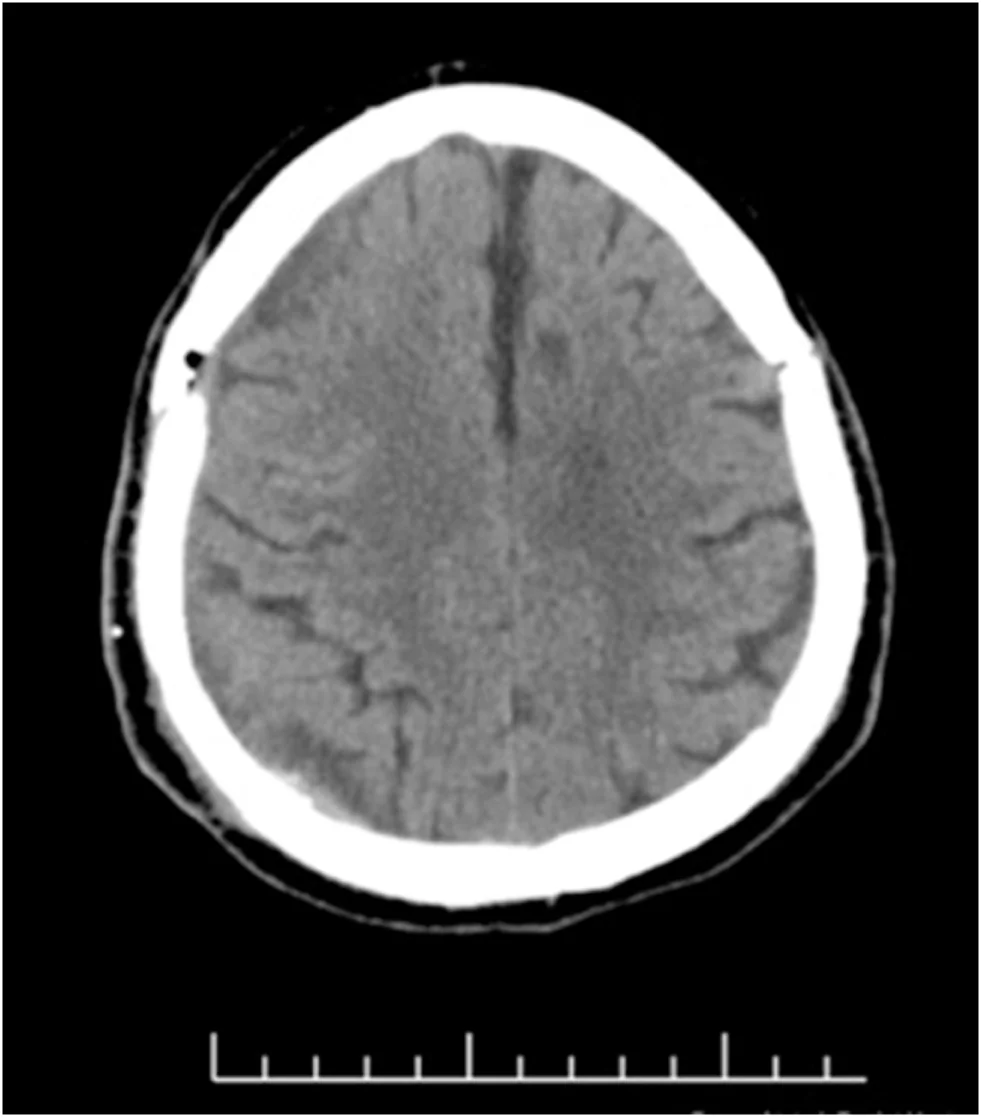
Three-month follow-up CT scan confirming the absence of recurrence or residual disease.
